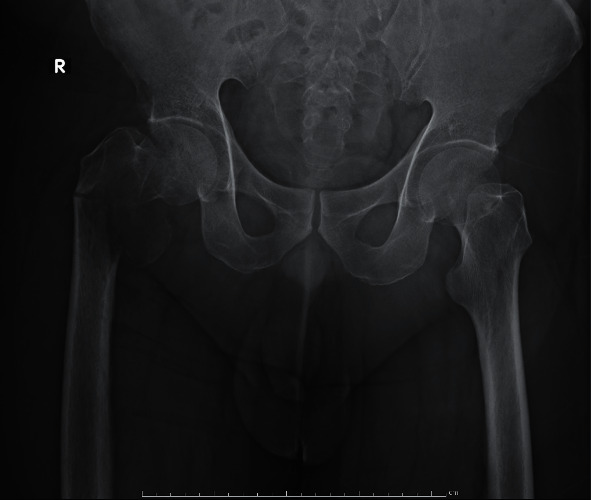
Plain radiograph revealed osteolytic lesion at right proximal femur with minimally displaced pathological fracture at the intertrochanteric region.
